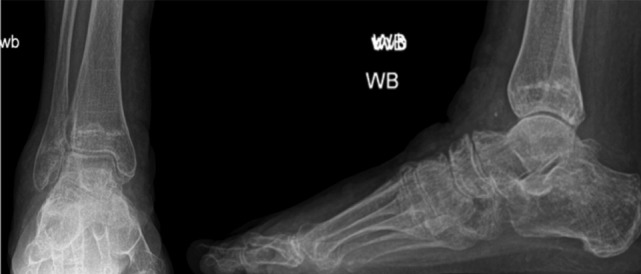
Plain weight-bearing radiographs of foot and ankle showing normal hind foot alignment.
